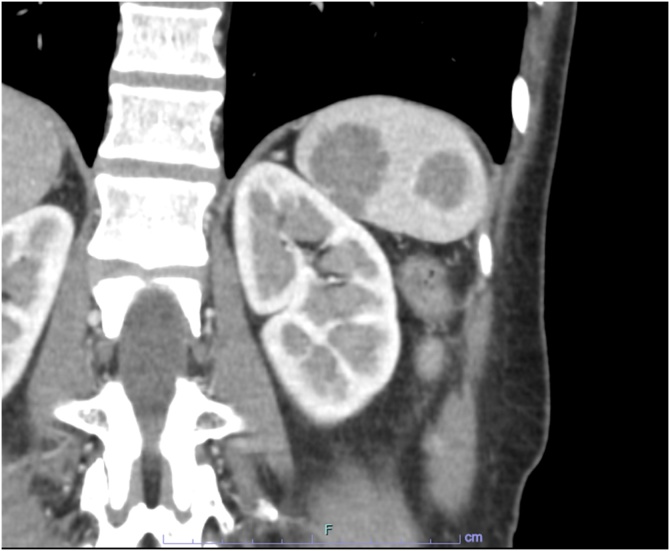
Abdominal enhanced computed tomography showing multiple hypoechoic nodules in the spleen.
